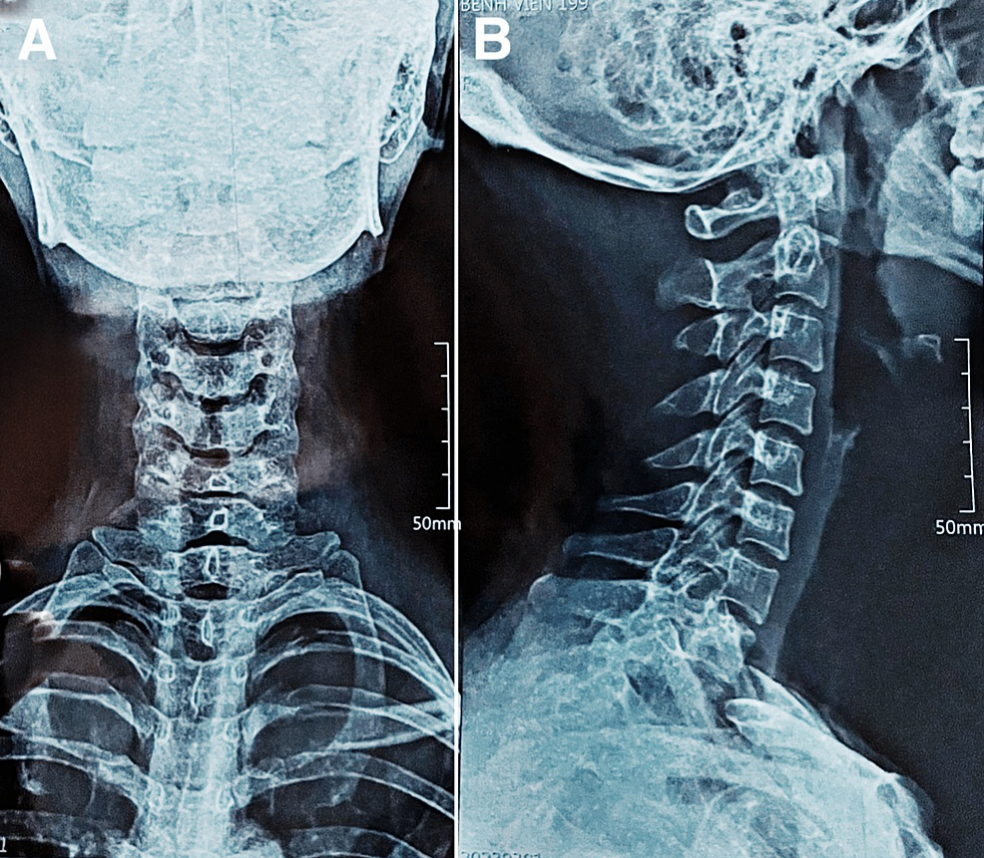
Initial cervical radiographs with unremarkable frontal (A) and sagittal (B) views.
